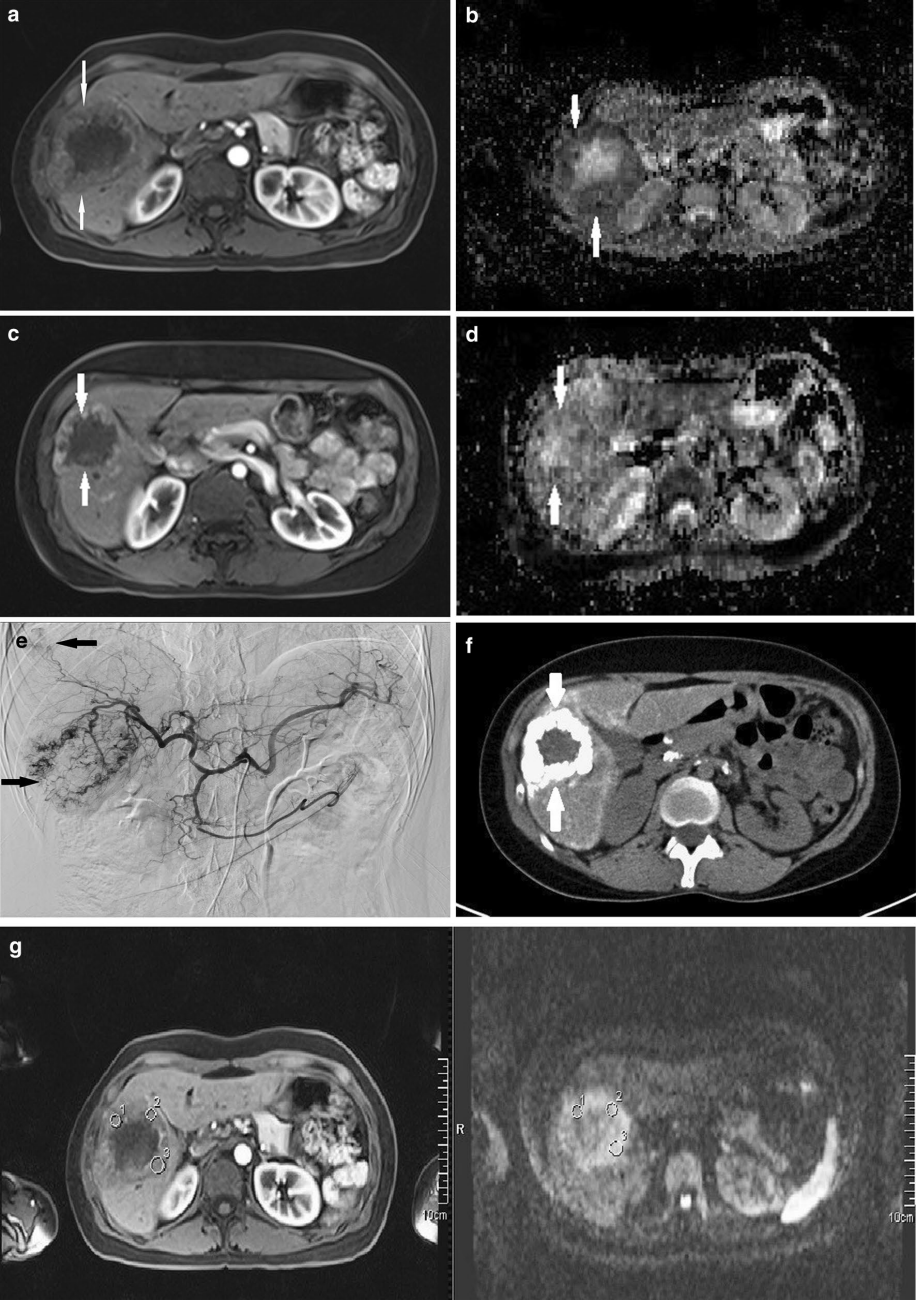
MRI in a representative 40-year-old participant with hepatitis B and cirrhosis who presented with two HCC lesions in segment 7 and segment 8. a Axial acquired contrast-enhanced T1-weighted MRI at baseline showing an HCC-typical lesion in segment 7 with central necrosis. b Baseline ADC-map demonstrating low ADC-values in the tumor mass and high signal in the necrotic area compared to normal ADC-values. c Axial contrast-enhanced T1-weighted MRI performed following the third TACE + DSM showing a RECIST partial response with an increase in size of the necrotic area. d ADC-map post-study completion showing increased ADC-values in the tumor mass as a predictor of therapy response. Values in the necrotic area remain increased. e Angiography demonstrating typical HCC hypervascularization with additional segment 8 hypervascularity of the second lesion. f Computed tomography following a 4-week-interval after the first TACE + DSM demonstrating prolonged Lipiodol uptake in the HCC lesion. g A post-contrast T1 sequence was linked to the ADC map for delineation of ROIs on the higher spatial resolution T1 images
